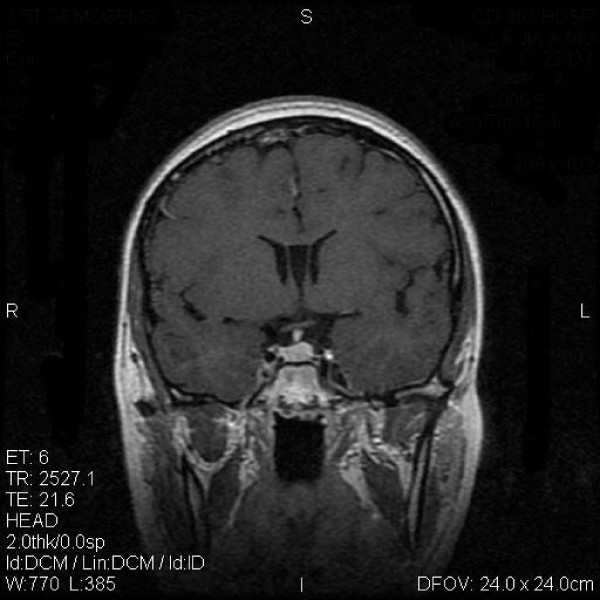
Typical MRI scan changes in prolactinomas adenoma. An enhancing mass lesion is seen in the sella turcia under the T1-weighted postcontrast MRI scan performed 1 year after MASEP GKRS, but the volume of the mass had collapsed for more than 50%. Patient 2's clinical symptom did improve. Her serum prolactin level came down to 14.5 ng/ml, and she got gestation and delivered a healthy baby recently.
